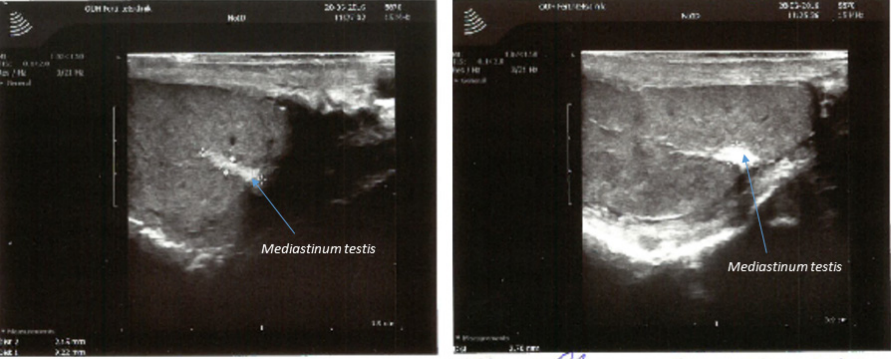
In normal testicles hyperechogenic, wedge‐shaped areas representing the *Mediastinum testis* are seen by ultrasonographic examination. [Colour figure can be viewed at wileyonlinelibrary.com].
